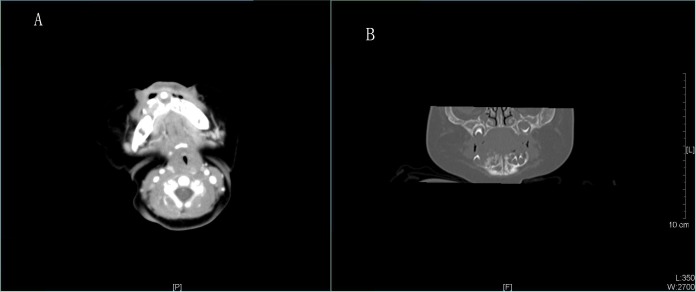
(A) Transverse and (B) coronal section computed tomography images of manibular melanotic neuroectodermal tumor of infancy of the right mandible.
